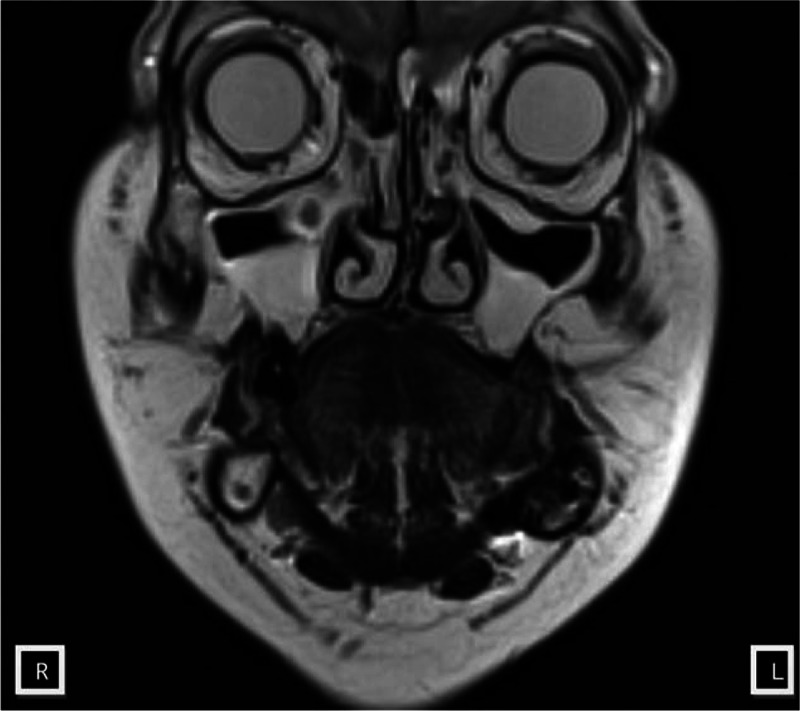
The tumor of the left mandibular angle in MRI scan. Bone loss filled with a tumor of irregular shape sized 40 × 30 × 18 mm in the left angle and mandibular ramus.
